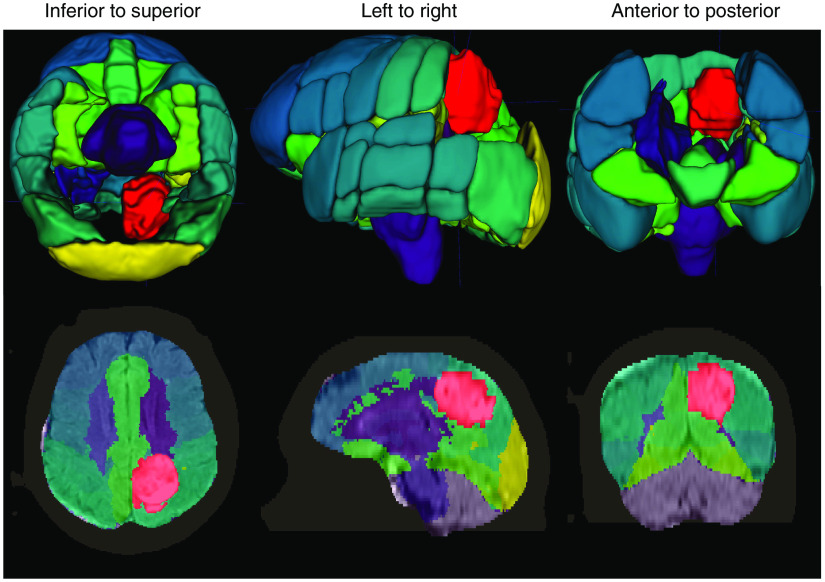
Example output from our DL system for automatic brain tumor segmentation. The system loads an MRI exam containing a T1-weighted postcontrast scan (T1c) and a FLAIR scan. Input from a wide range of MRI scanners and with varying scan parameters will work. We designed the system to perform the following steps automatically, without additional input: (1) enhance the MRI scans to remove artifacts; (2) identify the brain within the MRI scan (strip the skull), even in the presence of significant pathology or surgical interventions; (3) segment the brain tumor; and (4) coregister the Harvard-Oxford probabilistic atlas to the brain. The last step is used for visualization purposes and is optional. In this image, the tumor is red. Other colors indicate various atlas regions. The top and bottom rows show 3D and 2D views of the output data, respectively. Several atlas regions in the vicinity of the tumor have been made transparent in the 3D view to aid tumor visualization.
